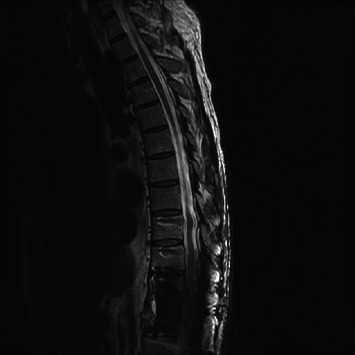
MR thoracic spine T2-weighted image 2 months postoperatively shows decompression of the spinal cord with instrumentation.
